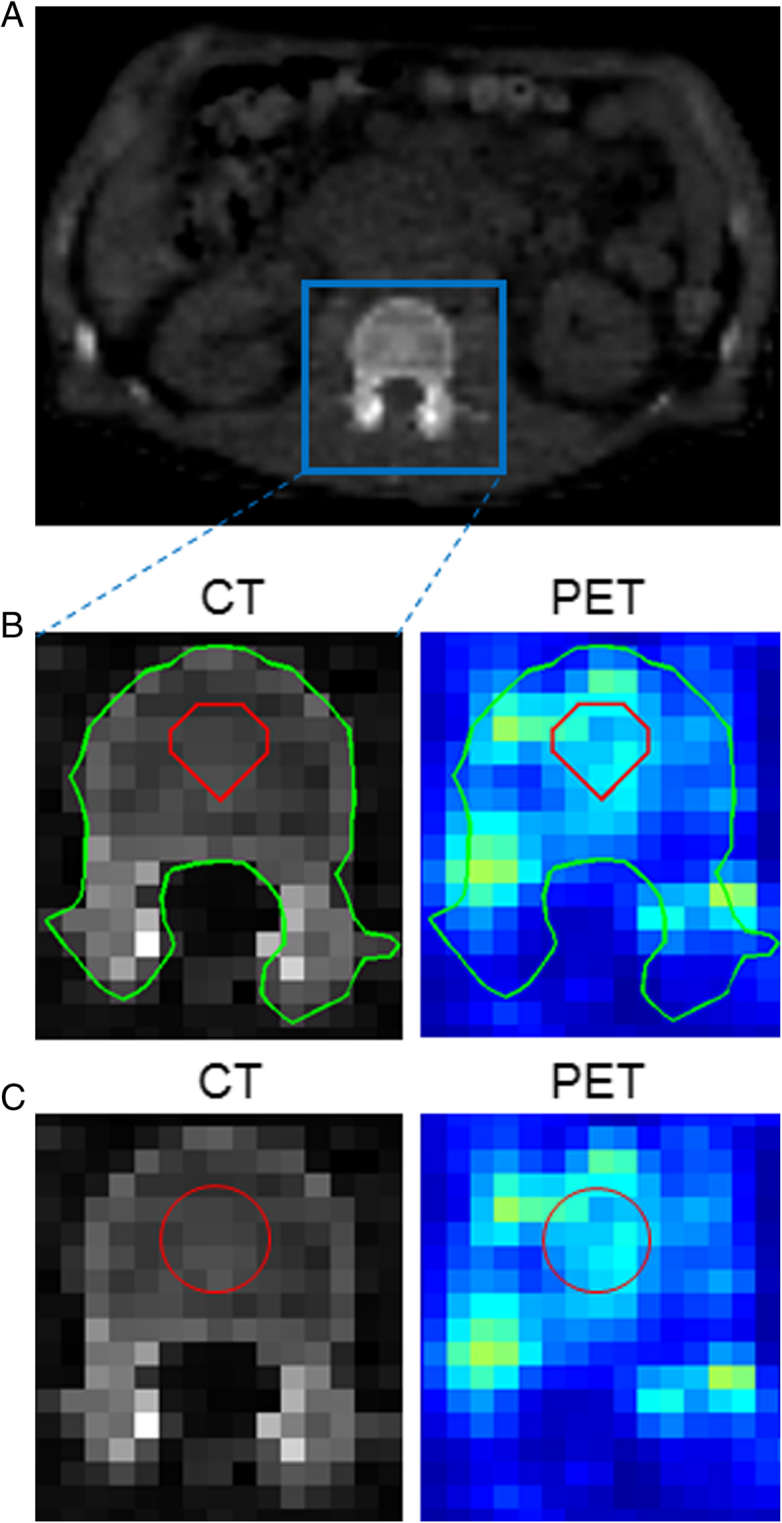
Use of a low dose CT image (a) to derive active (b) and manual (c) contours in one CT slice which are then projected onto the coregistered PET image to determine radioactivity concentration of the enclosed volumes of interest. In each CT slice, a loose region (c_1_—*blue*), enclosing the LV segment, was defined as the starting point of the active contour algorithm. In the next step, the active contour method was applied so as to identify the outer bone contour (c_2_—*green*) of the LV. Lastly, erosion of c_2_ was performed by sampling three pixels (vertically and horizontally) so as to exclude the compact bone from the LV segment, allowing for the creation of a contour that encompasses only the bone marrow component (c_3_—*red*)
